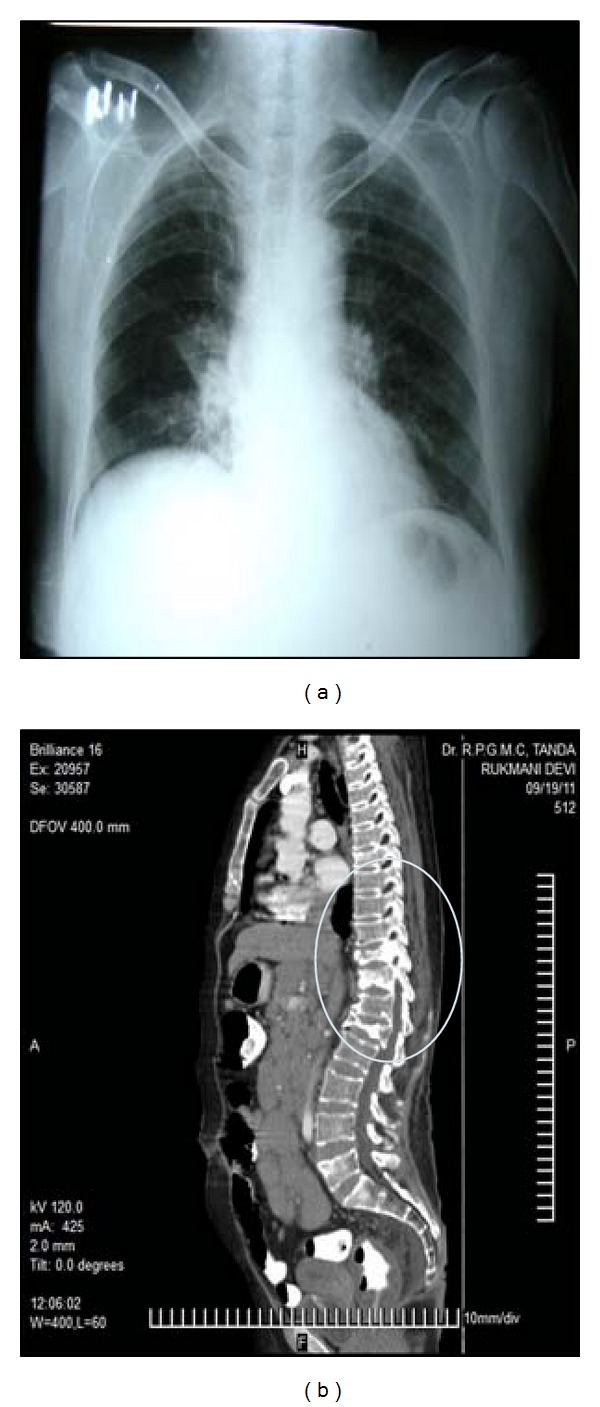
Segmental atelectasis in right parahilar region and bilateral hilar lymphadenopathy in X-ray chest (a). Vertebrae fractures of D10, D11, and L1 in CT scan (*encircled*) (b).
